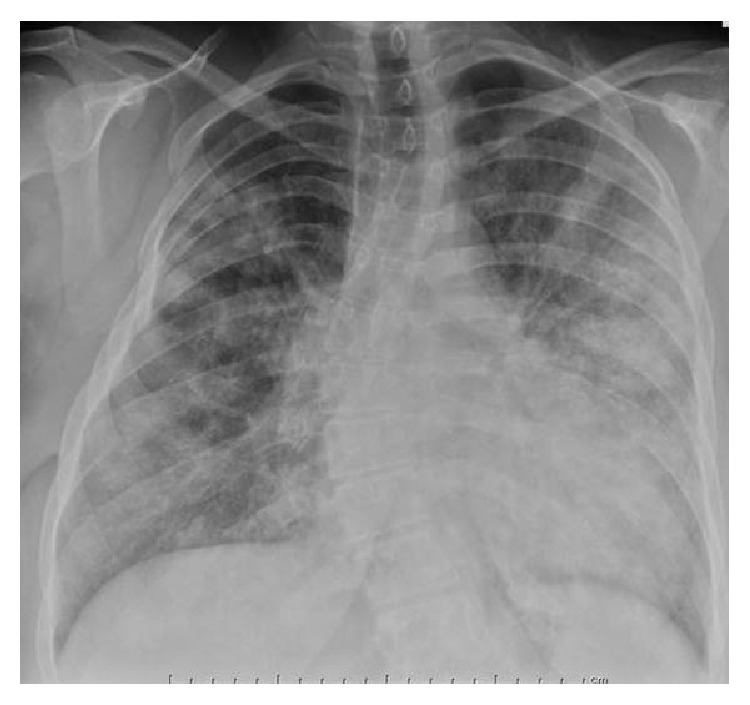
Showing the chest radiograph posteroanterior view. The cardiac silhouette is mildly enlarged but stable. The hilar structures are unremarkable. There are diffuse bilateral air space opacities. There is S-shaped thoracolumbar scoliosis.
